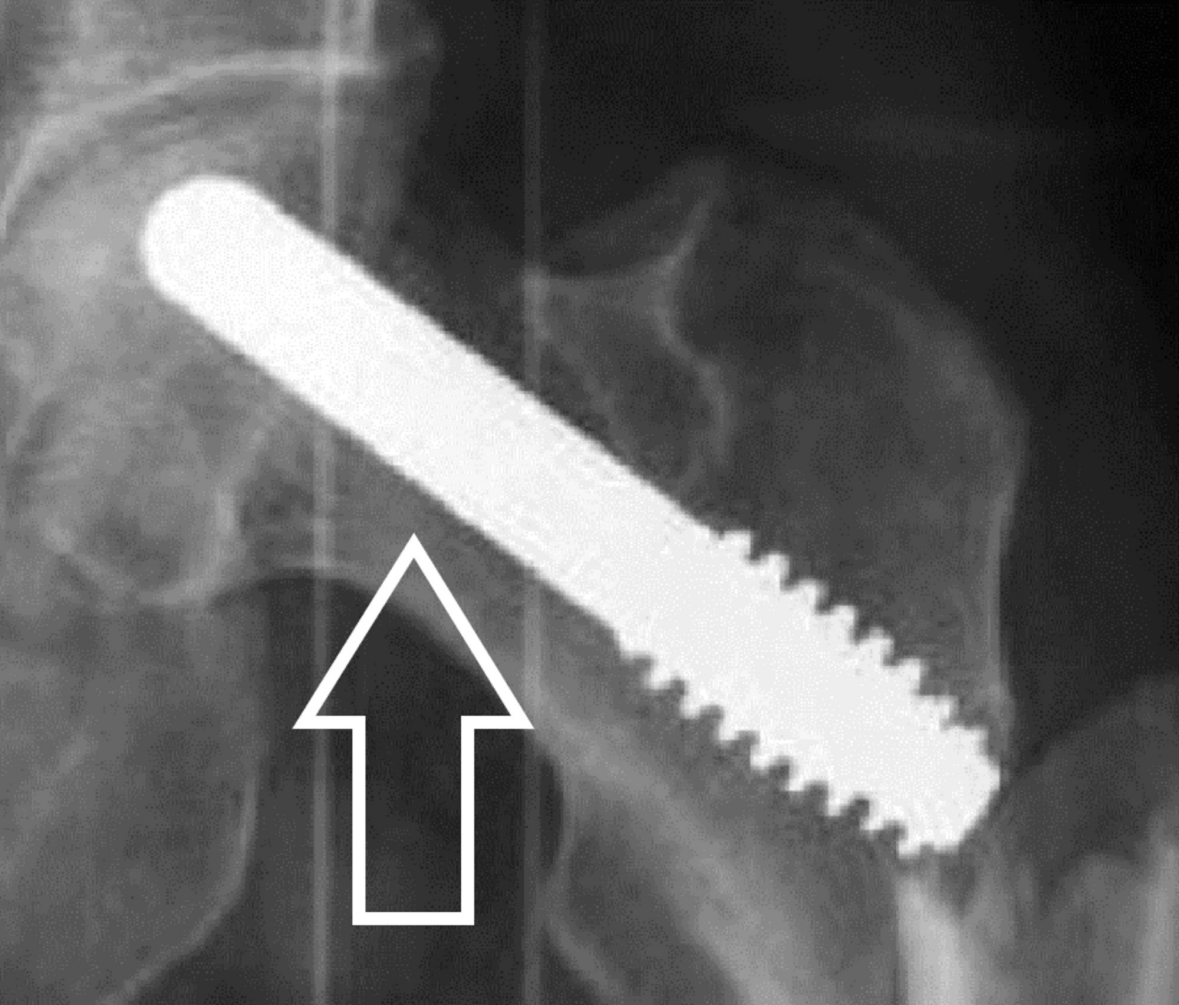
X-ray left hip with Tantalum rod Tantalum rod in vivo highlighted by an arrow.
